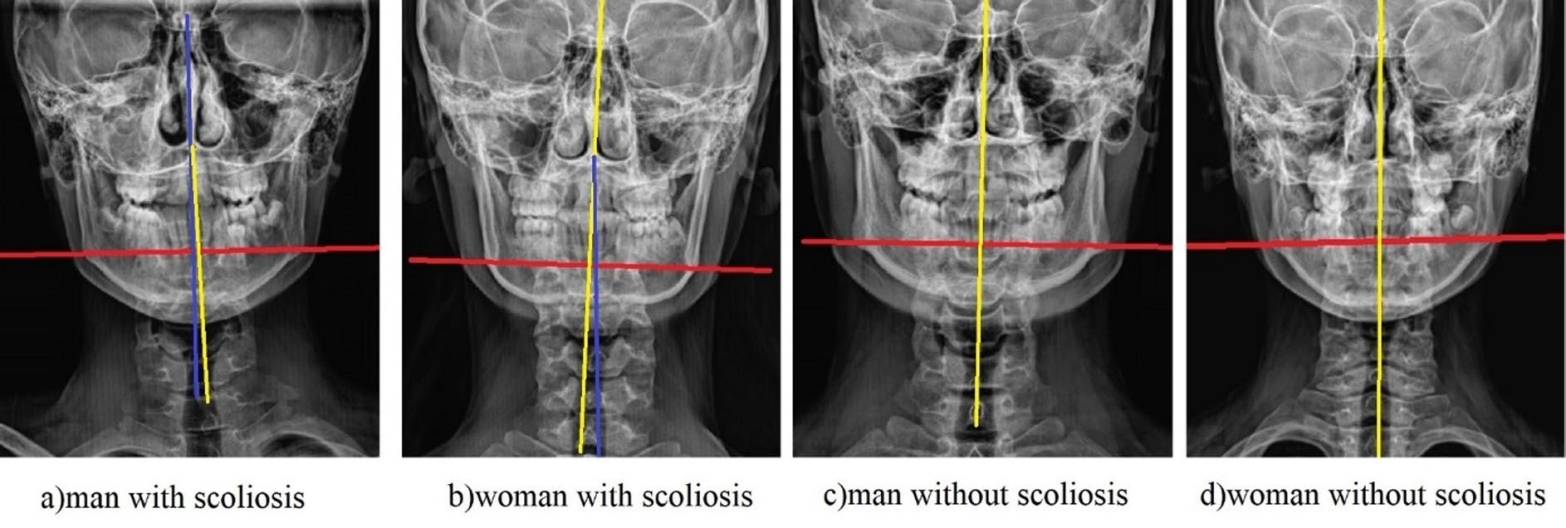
Method for quantitative assessment of facial asymmetry from posteroanterior cephalometric radiographs. The vertical yellow line represents the constructed cranial reference midline. The blue line represents the actual facial midline derived from manually placed landmarks (e.g., nasion, anterior nasal spine, and tip of the chin). The angle between these two lines (θ) was automatically calculated by a custom script written in Python (version 3.13). A screenshot of the script interface is provided in Fig. 2. This angle was defined as the ‘asymmetric angle score,’ which served as the primary quantitative measure of facial asymmetry.
